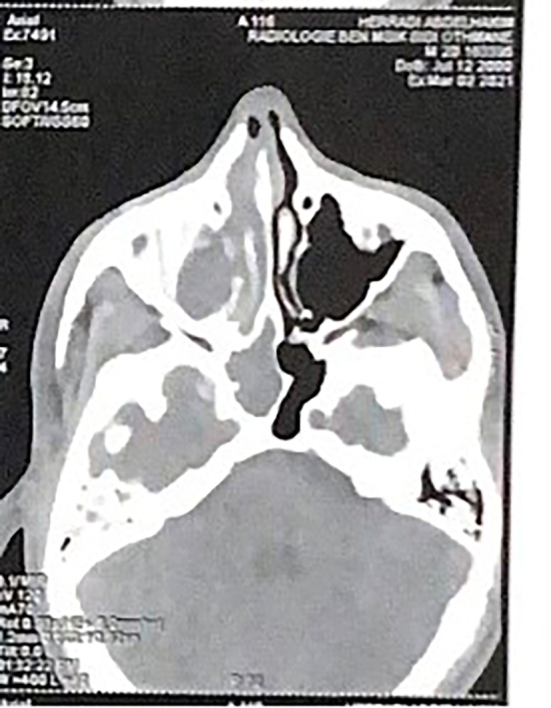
CT axial section showing an extensive right antrochoanal polyp.
